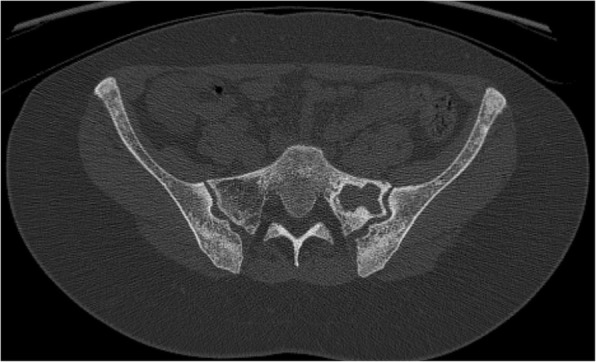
CT of the patient showed a well-corticated lucent lesion with patchy high density areas in the rest of the pelvic bone in keeping with previous rickets
